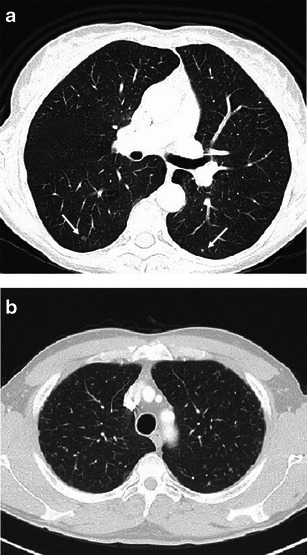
PLCH presenting with nodular features that mimic other diseases. a PLCH referred to metastasis: a 44-year-old woman, smoker, with a medical history of carcinoma of cervix uteri, treated with surgery and chemotherapy. A 3-mm collimated CT performed during oncological follow-up shows some faint micronodules in both lungs (*arrows*). Definitive diagnosis of PLCH obtained by VATS. b PLCH mimicking sarcoidosis: a 41-year-old man, smoker, with asymptomatic PLCH and skull involvement. Diagnosis obtained by skull biopsy. A 3-mm collimated CT shows numerous millimetric nodules. Nodules are mostly spread at the upper and middle lung fields in a peripheral location; few are subpleuric
